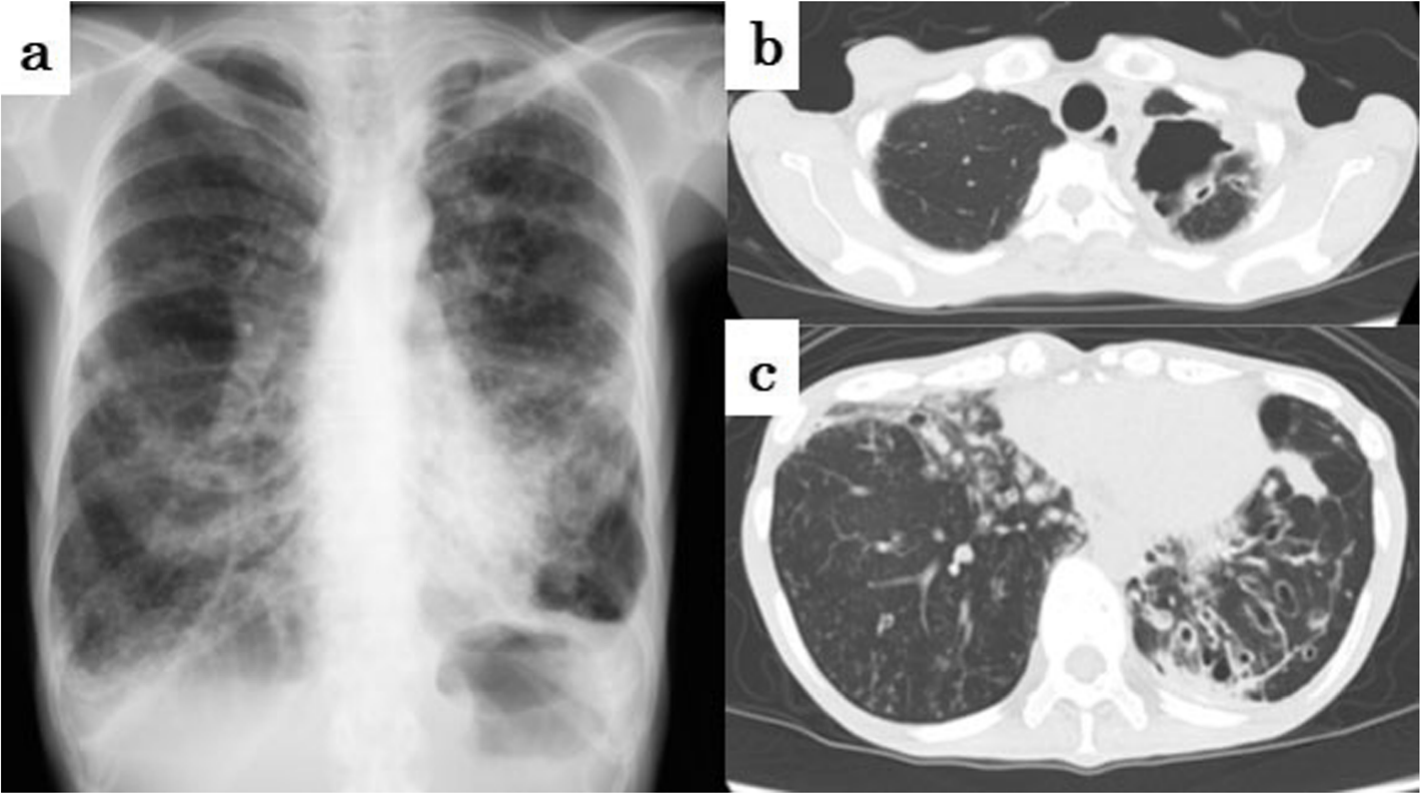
Chest images taken at the first visit at our institution in 2014. a Chest radiograph, b cavity lesion of the left lung apex on high-resolution computed tomography, and c left dominant bronchiectatic lesions on high-resolution computed tomography are shown
